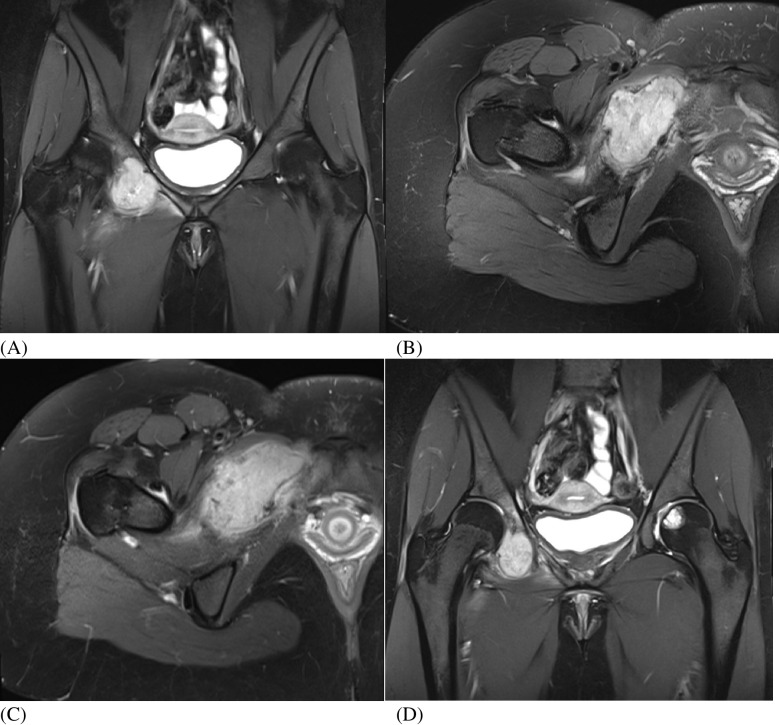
Right hip MRI (Siemens Healthineers MAGNETOM Skyra 3 T). A, B Coronal T2 FS Dixon_W (TR 3030 ms, TE 66 ms) and axial T2 FS (TR 3460 ms, TE 64 ms) images demonstrate a destructive mildly T2 hyperintense mass in the right superior pubic ramus and acetabulum with a large soft tissue component extending into the adjacent adductor muscles and hip joint. There is cortical breakthrough, and surrounding soft tissue, bone marrow, and periosteal edema. C Axial T1 FS Post (TR 804 ms, TE 24 ms, 7.33 mL Gadavist) images show a lobulated soft tissue mass, which demonstrates intense enhancement on T1 post-contrast imaging. D Coronal T2 FS Dixon_W (TR 303 ms, TE 66 ms) limited large field-of-view image reveals an additional T2 hyperintense lesion in the left femoral head epiphysis without significant surrounding bone marrow edema and intermediate signal peripheral rind (arrow)
